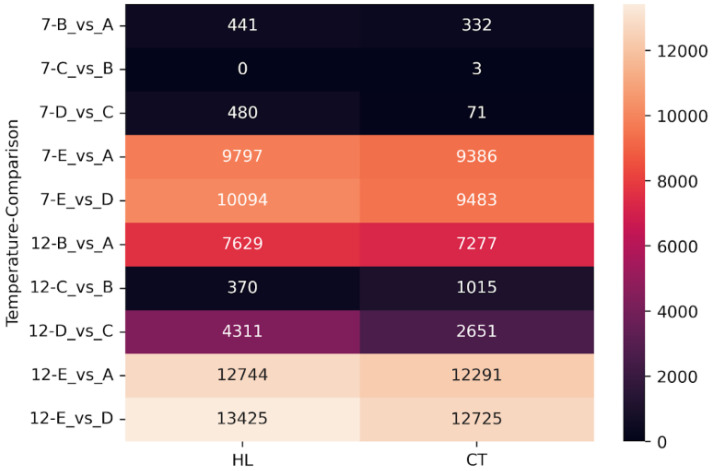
Numbers of DEGs for heat-loving (HL) and cold-tolerant (CT) cultivars.
